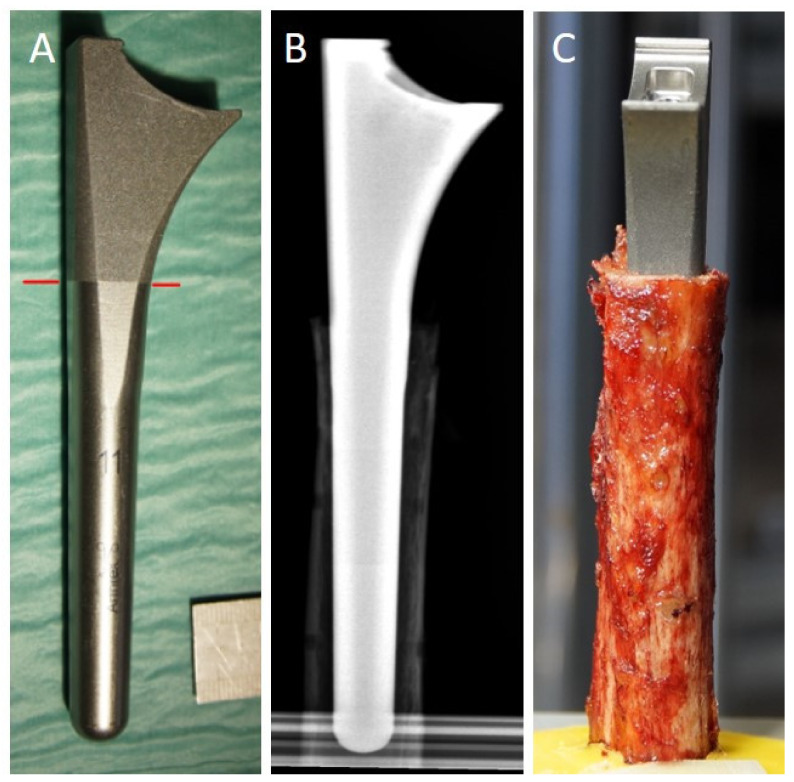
(A) Univers Revers stem (size 11), red line marks the junction between dia- and meta-physis. (B) X-ray prior to biomechanical testing confirming the correct stem size in relation to the humeral shaft. (C) Clinical picture prior to the start of testing confirming the correct position of the dia-/meta-physal junction in relation to the humeral shaft entrance.
